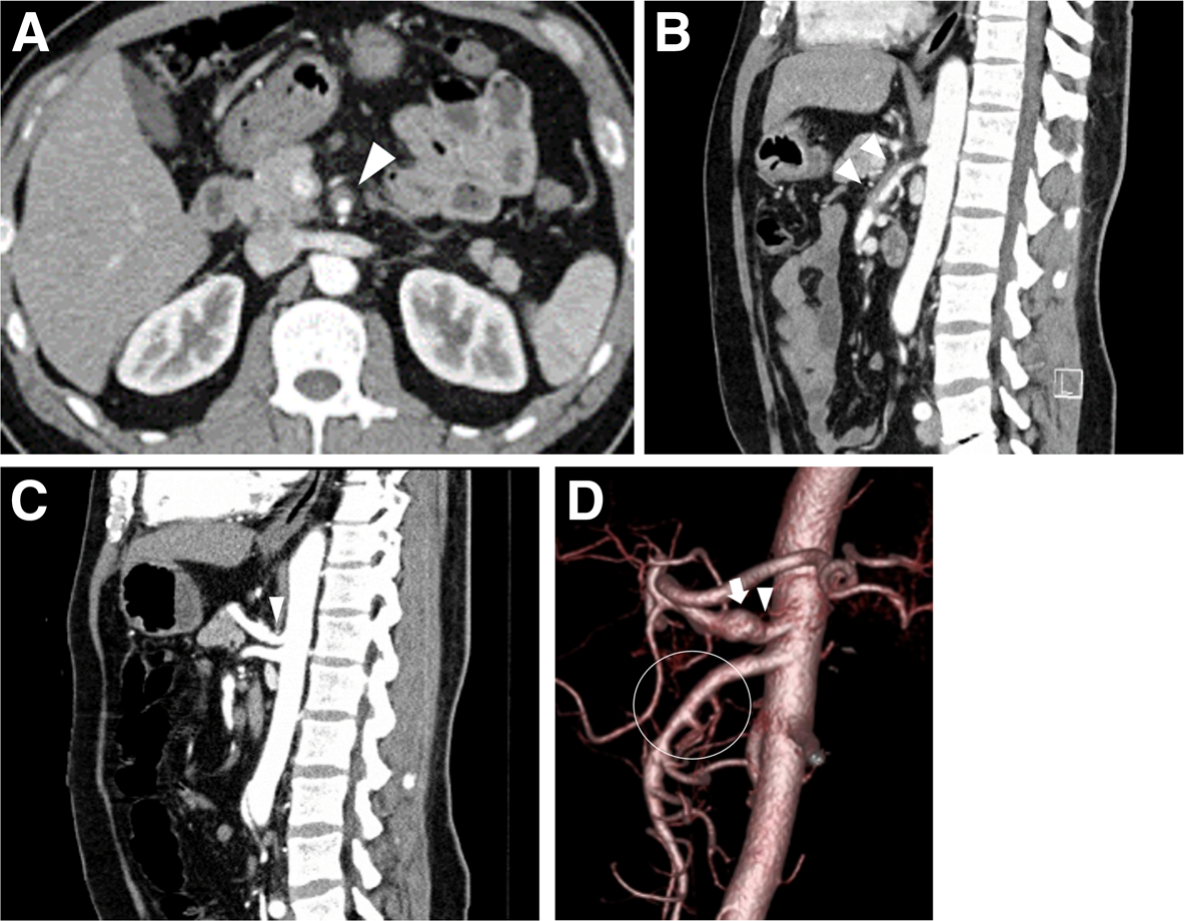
a, b Superior mesenteric artery dissection with the thrombosed false lumen is shown by arrow heads on an axial view (a) and a sagittal view (b) of contrast-enhanced CT. c Sagittal view of enhanced CT showing extrinsic compression of the root of celiac axis by the MAL (arrowhead). d 3D reconstruction of abdominal aortic angiotomography showing severe stenosis of the proximal segment of the celiac axis caused by extrinsic compression of the median arcuate ligament (arrow head) and poststenotic dilatation (arrow), and narrow true lumen of superior mesenteric artery(circle) from isolated dissection with a thrombosed false lumen
